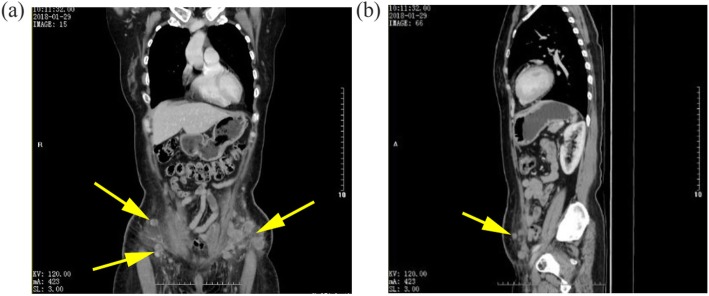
Contrasted CT scans showing multiple nodules and masses in the lower abdominal wall. a Coronal plane. b Sagittal plane
